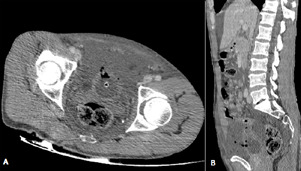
CT abdomen and pelvis revealing large amounts of free intraperitoneal fluid, leaking from the bladder through a small defect in the anterior bladder wall suggesting perforation, associated to intraperitoneal pelvic free air, with infiltrated perivesical fat, and empty bladder; A) axial view; B) sagittal reconstruction
